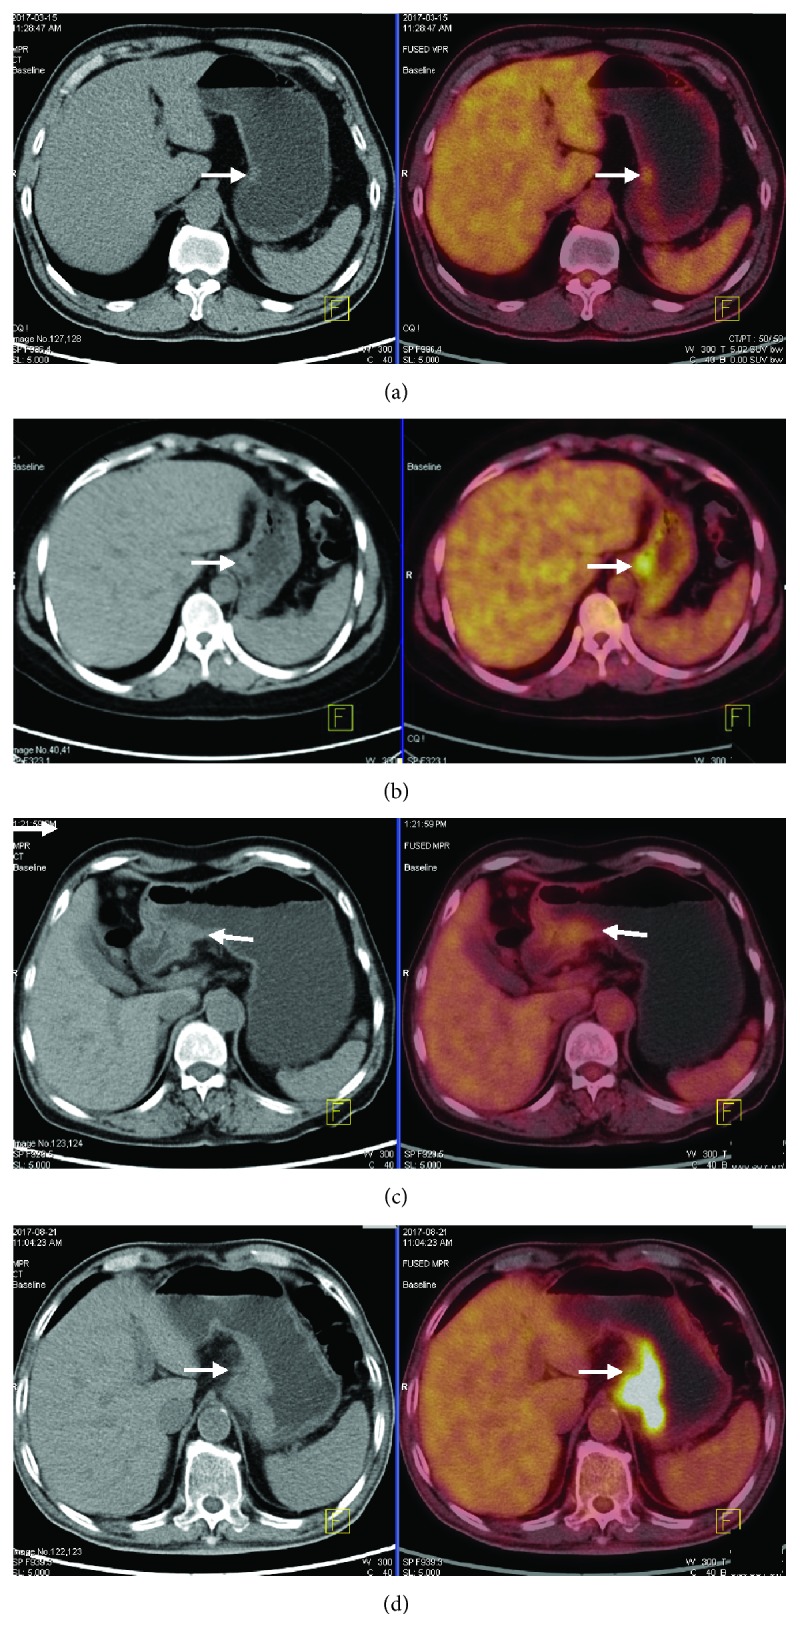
PET/CT images of T staging. (a) A 58-year-old man with gastric carcinoma pathologically diagnosed as stage T1; focal thickening of the gastric wall in the antrum with increased uptake tissues not exceeding the intermediate layer (arrow). (b) A 46-year-old woman with gastric carcinoma pathologically diagnosed as stage T2; focal thickening of the gastric wall in the cardia with increased uptake tissues exceeding the intermediate layer without infiltrating the whole thickened gastric wall (arrow). (c) A 70-year-old man with gastric carcinoma pathologically diagnosed as stage T3; focal thickening of the gastric wall in the antrum, the whole thickened gastric wall is infiltrated by the increased uptake tissues with a smooth and well-defined outer border (arrow). (d) A 74-year-old man with gastric carcinoma pathologically diagnosed as stage T4; thickening of the gastric wall in the cardia and fundus, the whole thickened gastric wall with an irregular outer border is infiltrated by the increased uptake tissues and transmural extension into perigastric fat (arrow).
